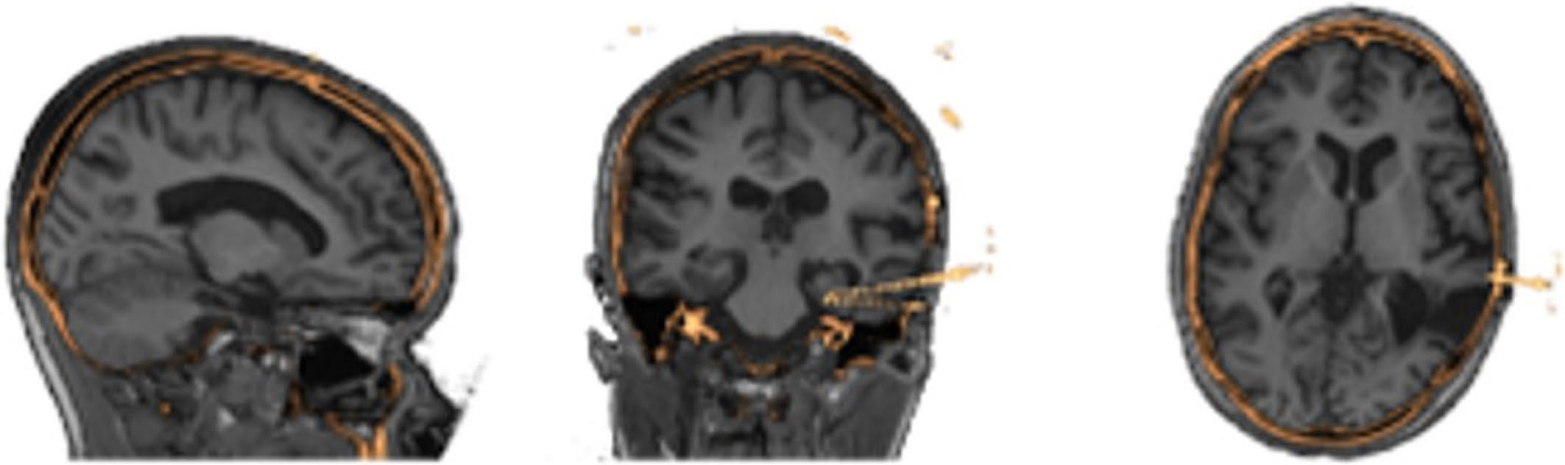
Results of image processing and registration as seen in three different views from the same patients’ MRI (grayscale) to their processed CT (gold) showing alignment of the two image spaces.
